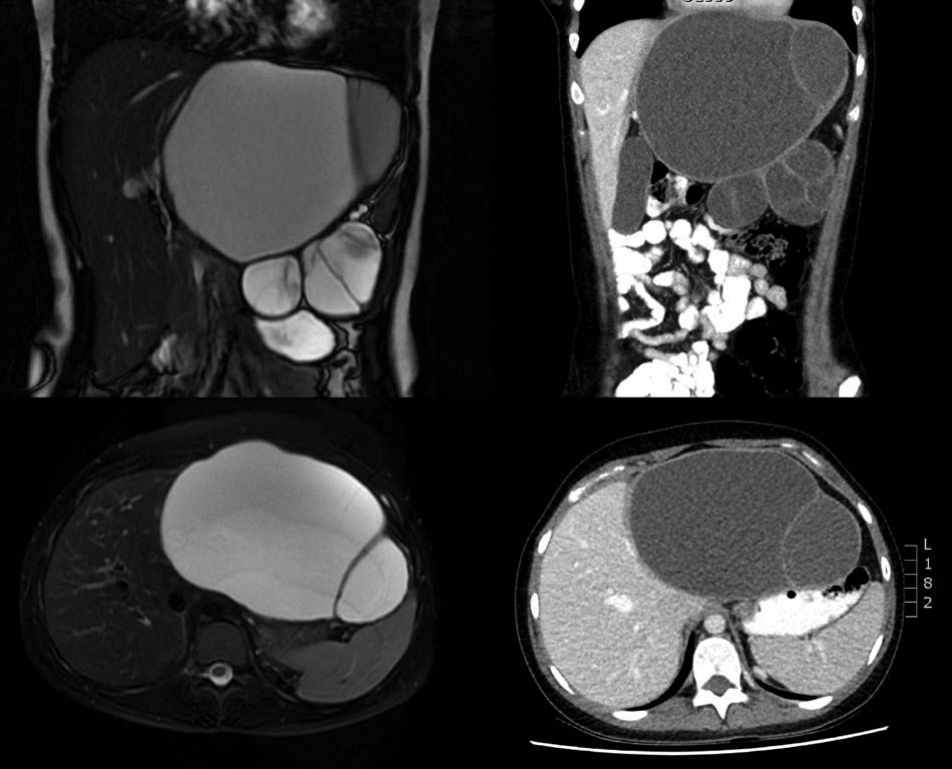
MRI (left) and CT (right) preoperative imaging.
